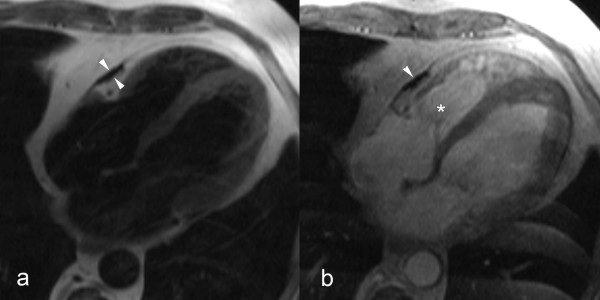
Focal constrictive pericarditis, axial T1-weighted spin-echo CMR (a) and axial cine CMR (b). Focal pericardial thickening (arrowheads) at the level of the right atrioventricular groove and basal part of the free wall of the right ventricle with focal compression of right ventricular cavity (*). The very low signal intensity of the focally thickened pericardium on T1-weighted spin-echo CMR as well cine CMR should alert one to look for calcification of the pericardium.
